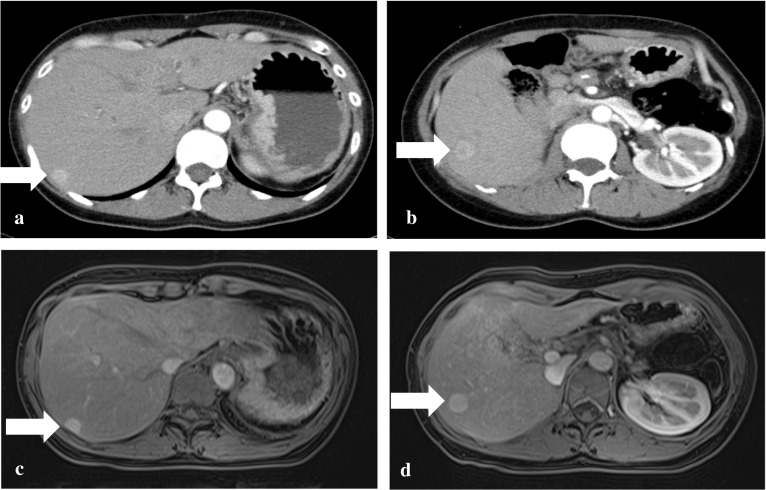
a, b Contrast-enhanced CT showed arterially hyper-enhanced lesions in liver segment VIII (a, arrow) and VI (b, arrow). c, d Gd-EOB-DTPA-enhanced MRI showed arterially hyper-enhanced lesions in liver segment VIII (c, arrow) and VI (d, arrow). *CT* computed tomography, *Gd-EOB-DTPA* gadolinium ethoxybenzyl diethylenetriamine penta-acetic acid, *MRI* magnetic resonance imaging
